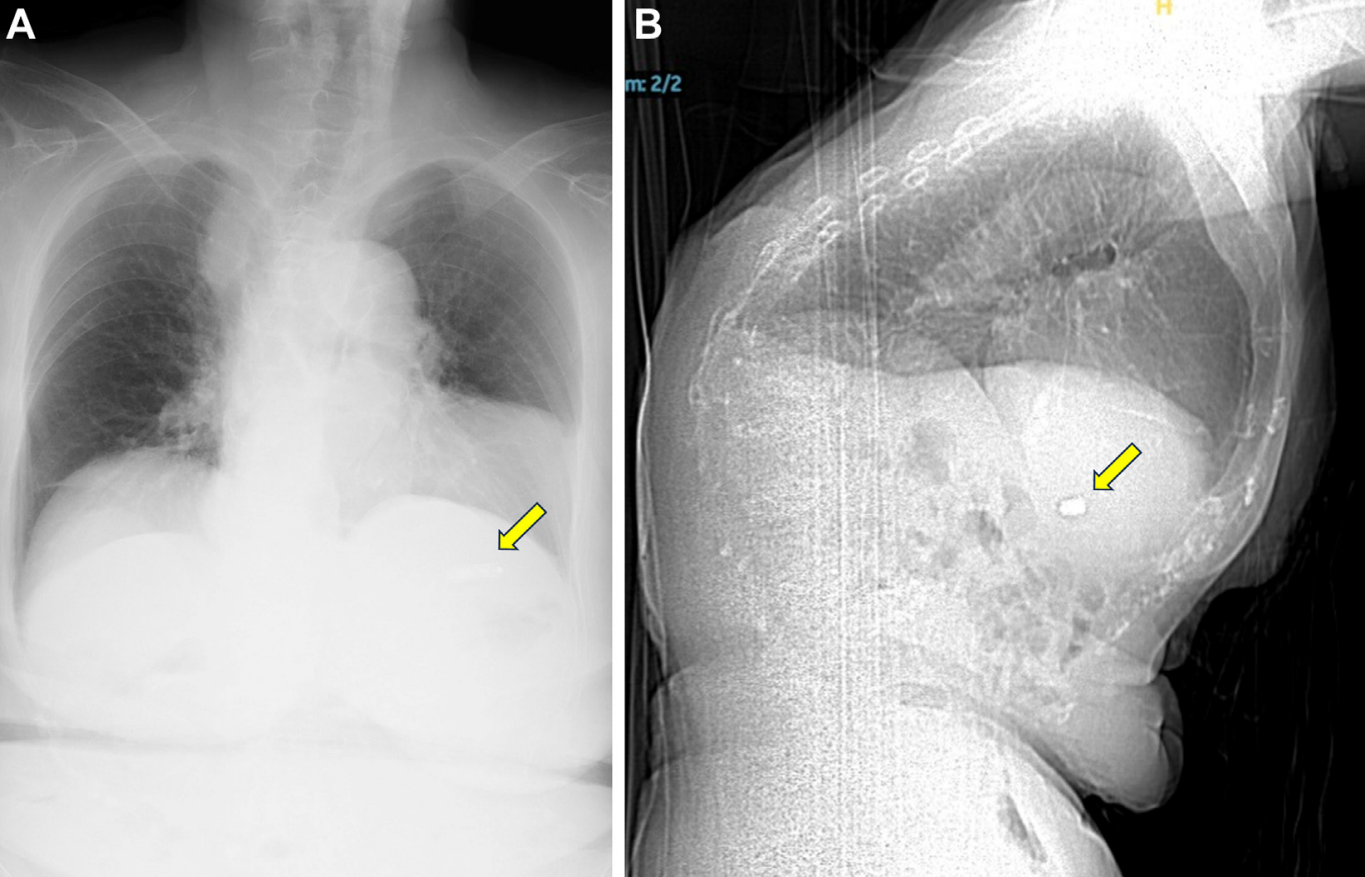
Radiography and Computed Tomography (A) Chest radiography and (B) sagittal-view computed tomography image showing anterior displacement of the heart caused by the barrel chest deformity and leadless pacemaker placement (yellow arrows).
